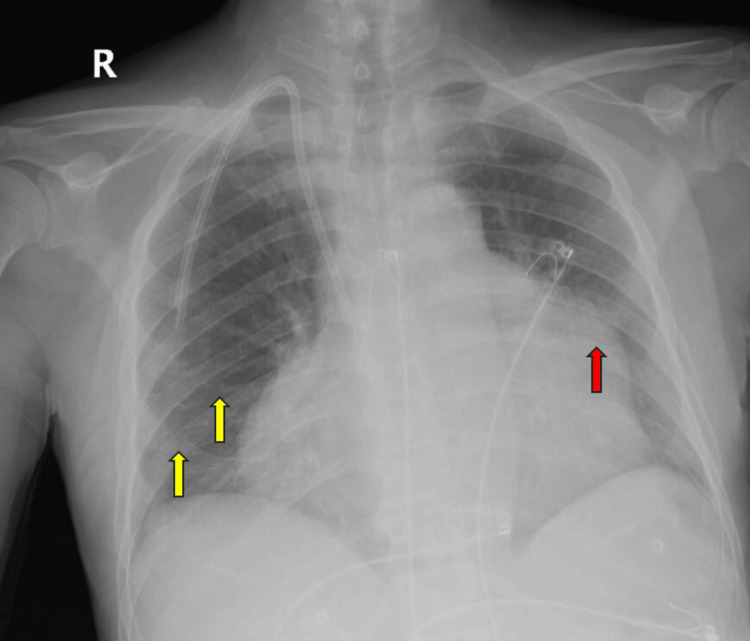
Chest X-ray showing bilateral vascular congestions (yellow arrows) and water-bottle-shaped heart (red arrow).
